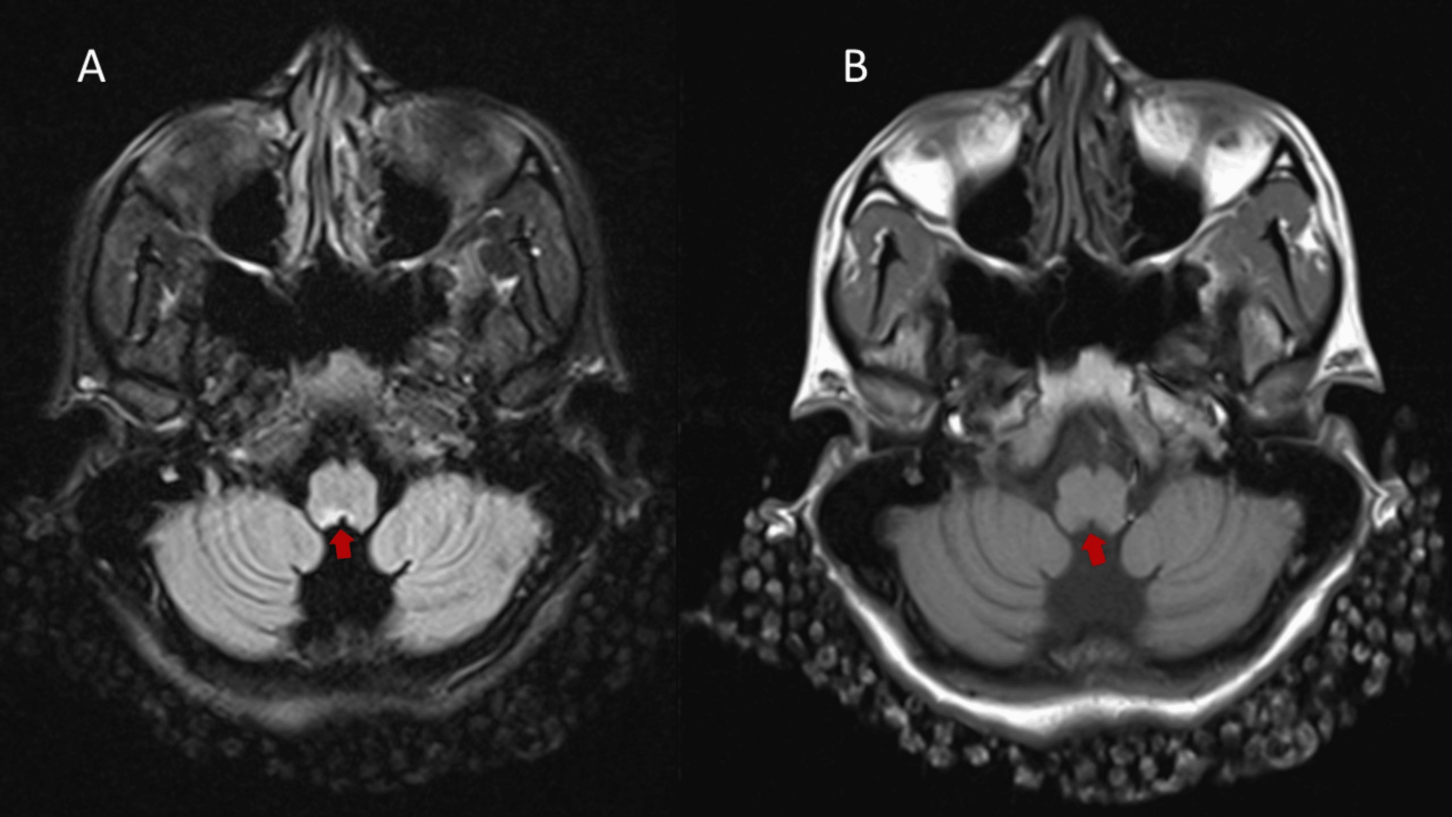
(A) T2/fluid-attenuated inversion recovery (FLAIR) hyperintensity at the right greater than left dorsal medulla. (B) T1 hypointensity at the right greater than left dorsal medulla. The arrows point to the lesion in the dorsal medulla, emphasizing the right.
